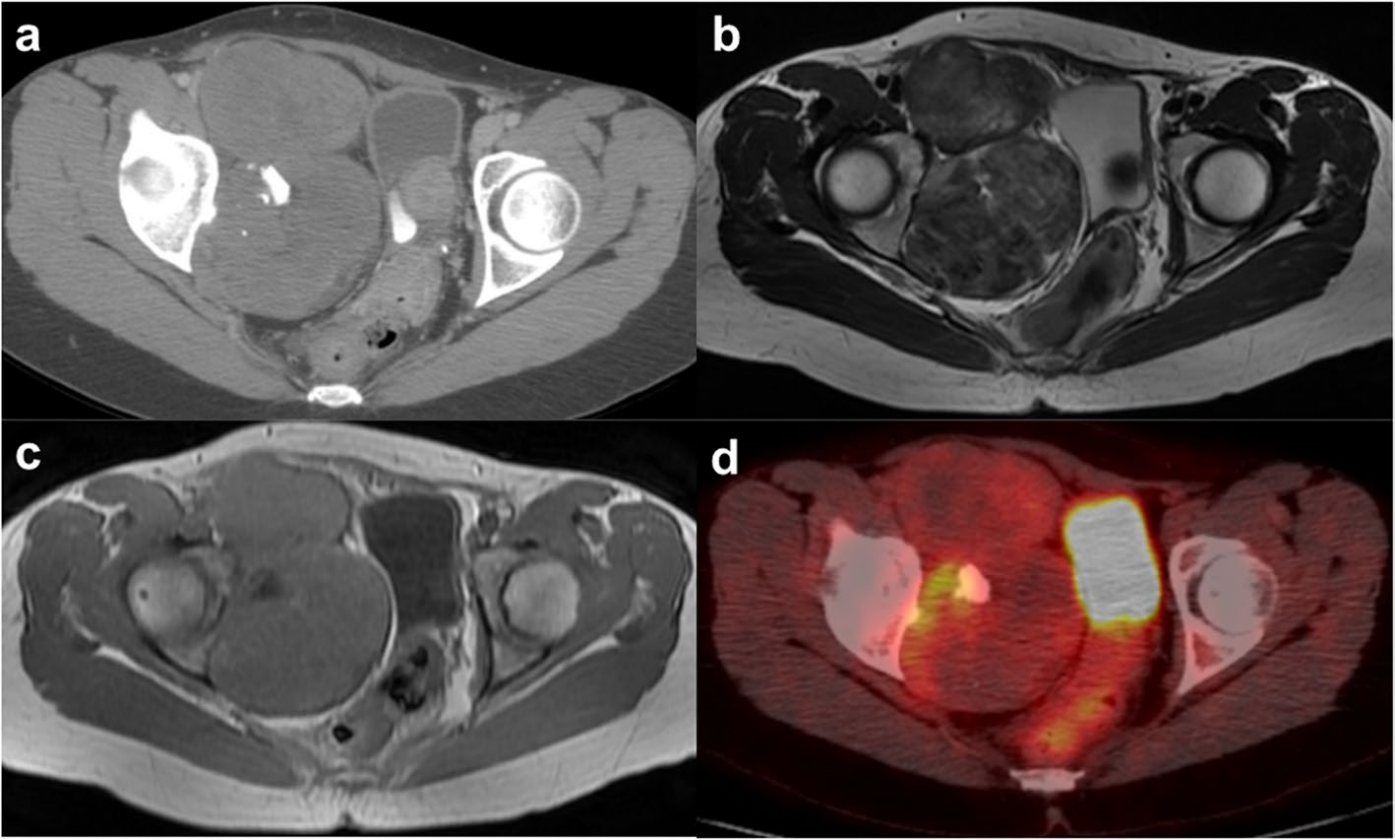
Radiologic image findings before the first surgery. Computed tomography (CT) showing a multilocular mass with calcification in the pelvic cavity (a). Magnetic resonance imaging (MRI) demonstrating iso-signal intensity on T1 weighted images (b) and hypo- and iso-signal intensity on T2 weighted images (c). ^18^Fluorine-labeled fluorodeoxyglucose–positron emission tomography showing an increased uptake of ^18^ F-fluorodeoxyglucose in the calcified part (SUV = 6.5) while no increased uptake is seen in other lesions (d)
